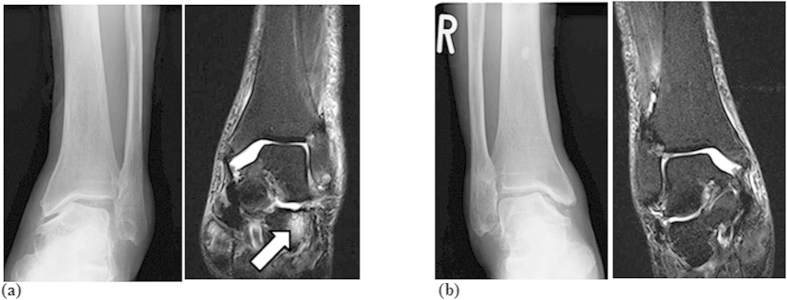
(a) Frontal view plain radiograph (left panel) demonstrated radiographic OA of KL grade IV. A frontal view (right panel) T1W MR image indicated bone signal changes along the talocalcaneal joint. (b) Frontal view plain radiograph (left panel) depicted radiographic OA of KL grade III. A frontal view (right panel) T1W MR image showed no bone signal changes along the talocalcaneal joint.
